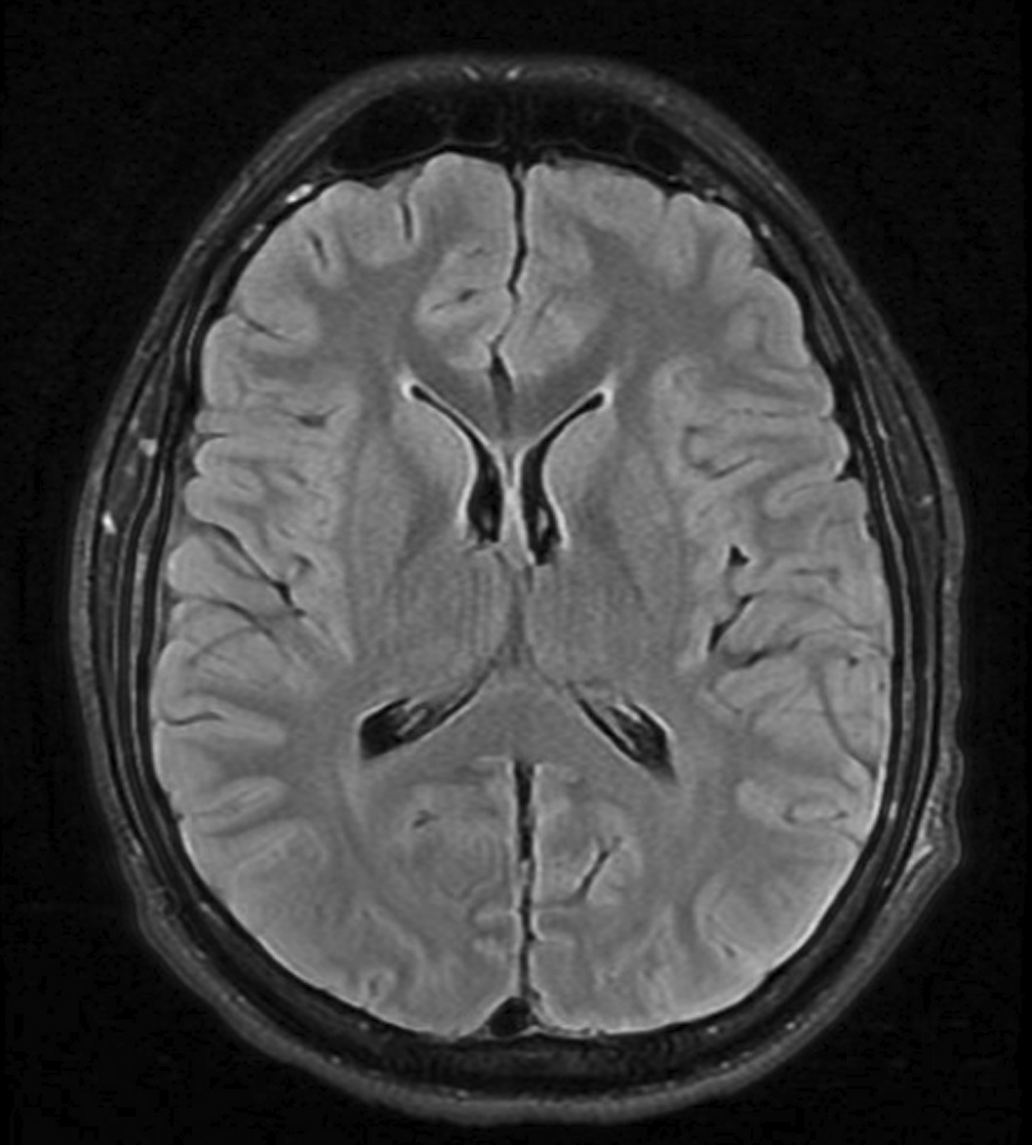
MRI head T2 FLAIR on axial view shows normal parenchyma and ventricles with no extra‐axial fluid collection, mass lesion or mass effect.
